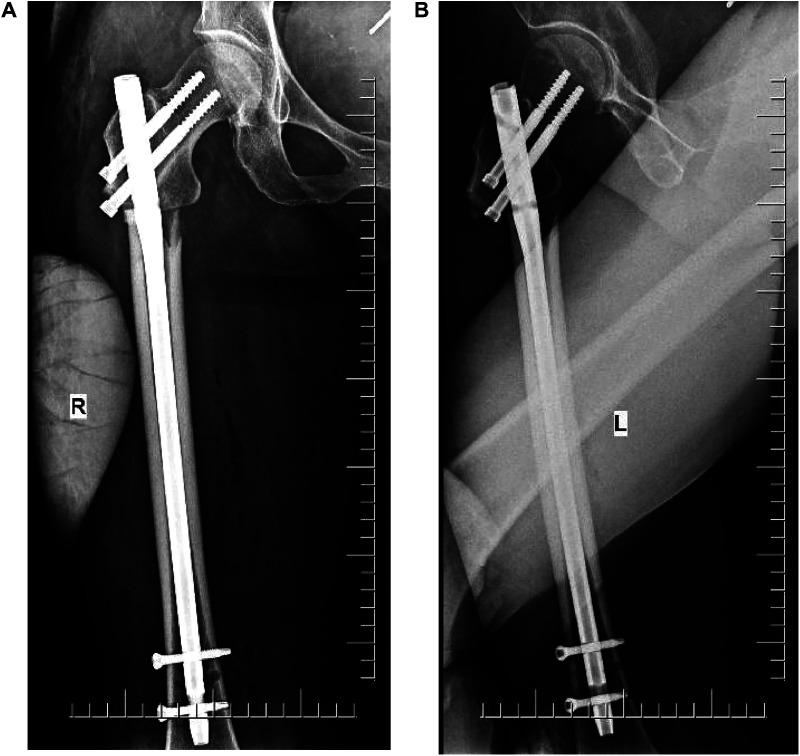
(A,B) X-ray (4 days after surgery). The lateral end of the fragment shows a displacement.
